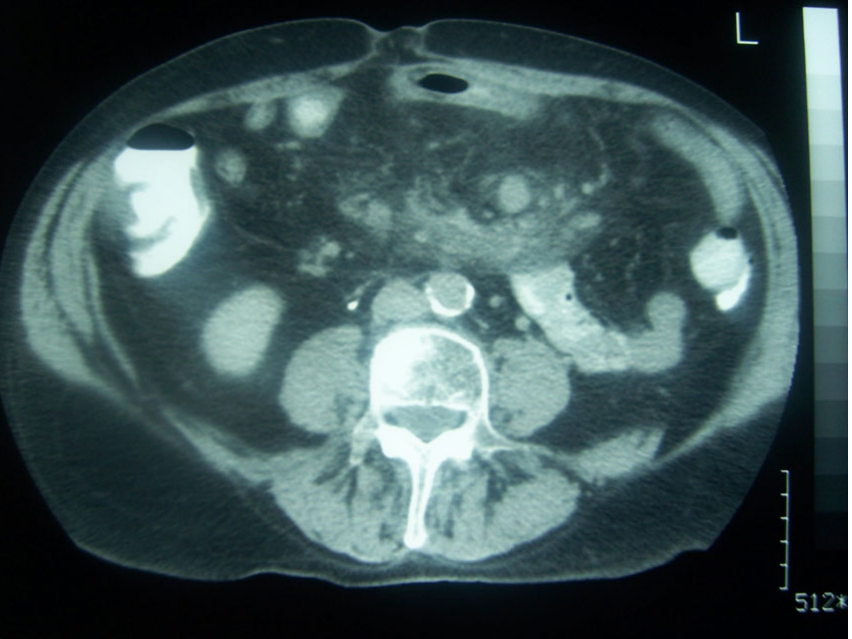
CT scan of the abdomen showed a mass involving the mesentery at the edge of which lays a loop of small intestine with thickened wall.
